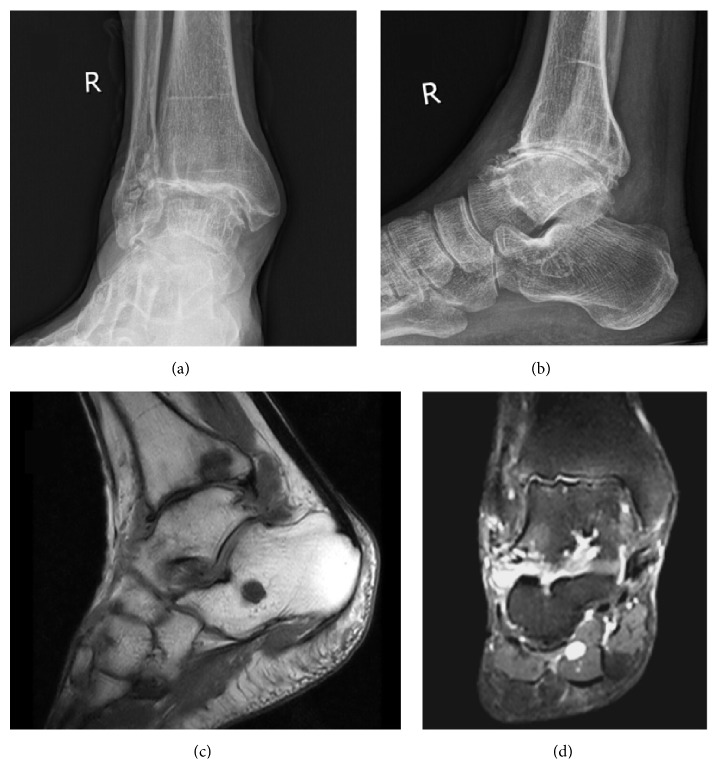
Preoperative imageological examination suggesting end-stage traumatic arthritis in the right ankle. (a) Preoperative radiograph of the ankle joint (anteroposterior view). (b) Preoperative radiograph of the ankle joint (lateral view). (c) Preoperative MRI of the ankle joint (lateral view). (d) Preoperative MRI of the ankle joint (anteroposterior view).
